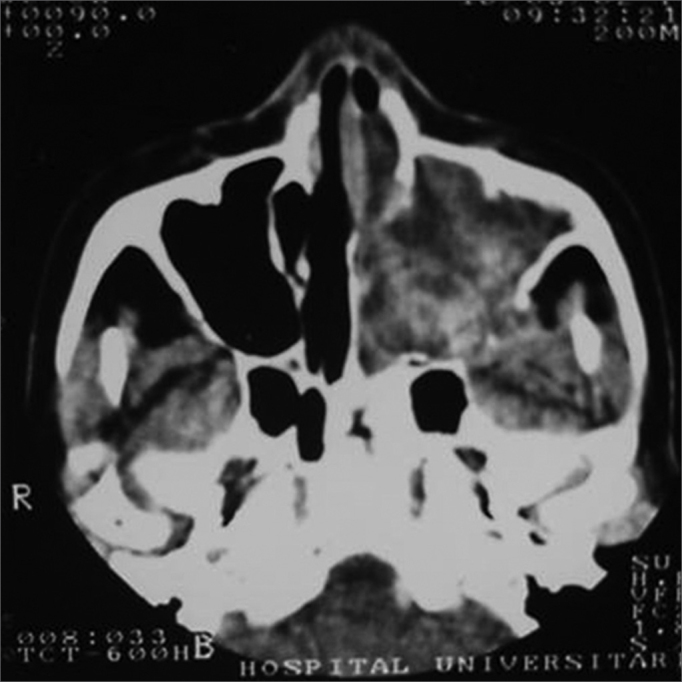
CT, axial slice, soft tissue window.
